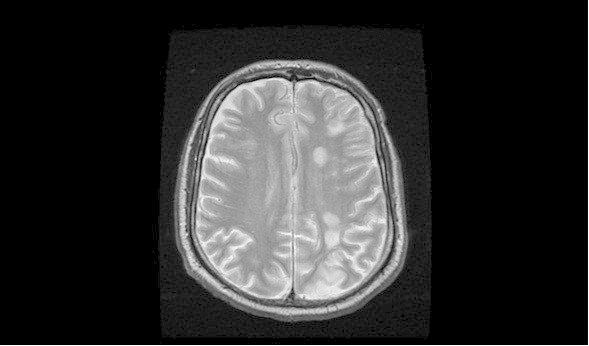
This axial T2-weighted MRI shows widespread bilateral sulcal FLAIR hyperintensity, which is consistent with SAH.
